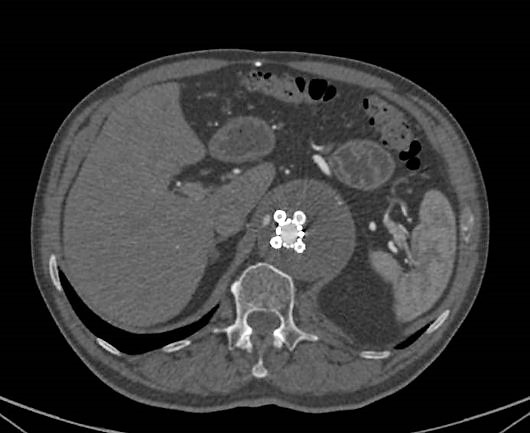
In CTA cross-section the docking station resembles the revolving
